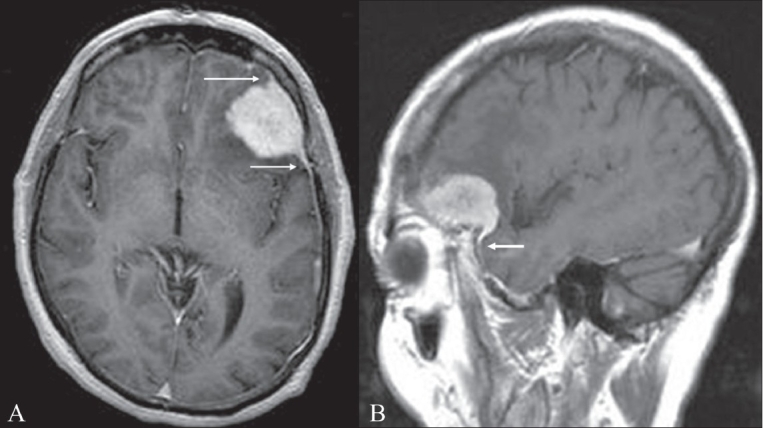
Dural tail sign. Axial (A) and sagittal (B) contrast-enhanced MRI images of the brain show an avidly enhancing lesion in the left frontal region. The lesion is dural based and there is thickening and enhancement of the dura, which tapers away from the mass (arrows). This thickening and enhancement of the dura adjacent to the mass is the ‘dural tail sign’ that is seen in meningiomas
